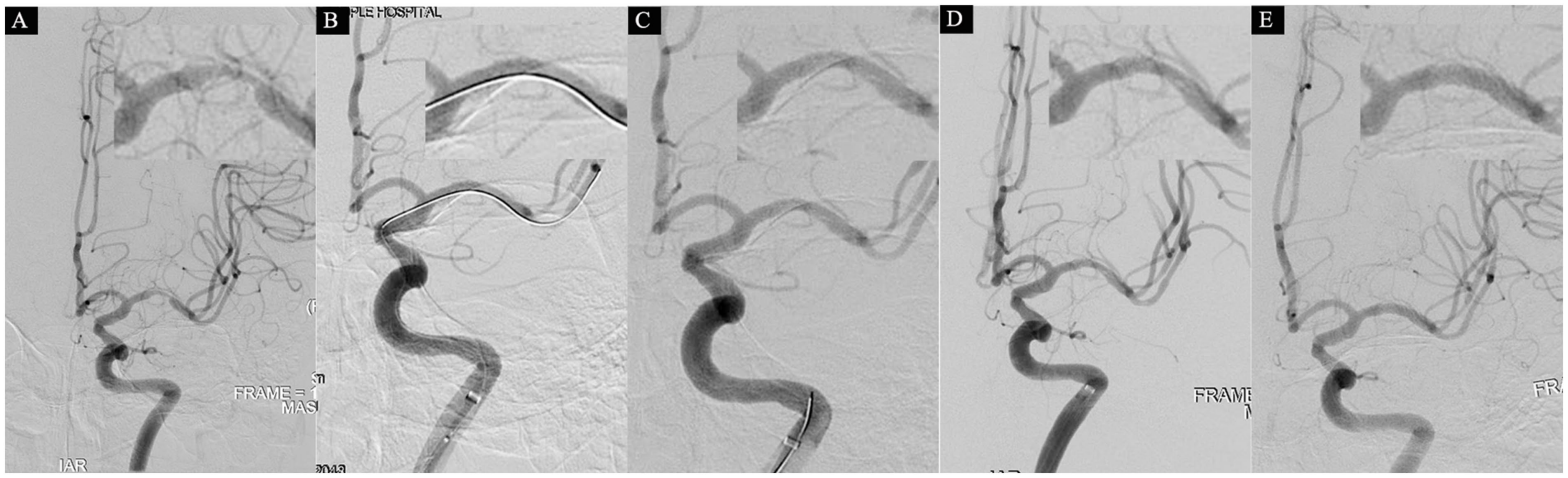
A 75-year-old male who was admitted on June 28, 2021, with a 22-day history of right-sided limb weakness. At admission, the NIHSS score was 0, and head MRI showed no evidence of acute infarction, leading to a diagnosis of transient ischemic attack (TIA). Subsequent cerebral angiography on June 30 revealed severe stenosis in the M1 segment of the right middle cerebral artery (MCA), characterized by a proximal lumen of 2.8 mm, the narrowest part at 0.6 mm, and a stenosis degree of 79% (A). An initial attempt at dilation was made using a 2.0 × 15 mm balloon, resulting in satisfactory reconstruction of the stenosis (B). However, upon withdrawal of the microguidewire for a follow-up angiogram, an unexpected dissection in the MCA was observed (C). After a 10-min observation, a subsequent angiogram indicated a more significant dissection, along with sluggish blood flow in the distal MCA (D), eTICI grade 2c. Consequently, a strategic decision was made to deploy a 3.0 × 13 mm balloon-mounted stent (APOLLP, MicroPort, China). A follow-up angiogram demonstrated successful eTICI grade 3 (E).
